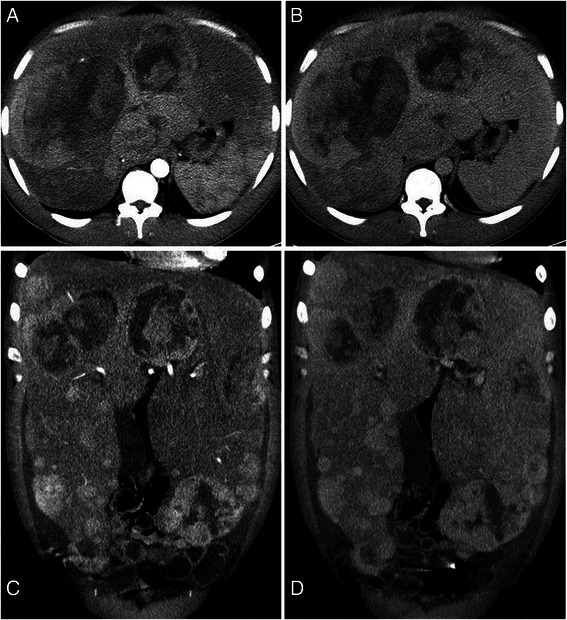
Contrast enhanced computed tomography in arterial (a,c) and portalvenous phase (b,d): Highly enlarged liver with curved borders secondary to multiple parenchymatous lesions throughout all liver segments, with arterial enhancement and no substantial wash-out during portalvenous phase. The large lesion in right lobe up to 16 cm appeared inhomogeneous with peripheral enhancement and non-enhancing, necrotic areas in the center. Intrahepatic vena cava and right liver vein were completely compressed by nodular tumors in the caudate lobe and the large lesion in the right lobe
